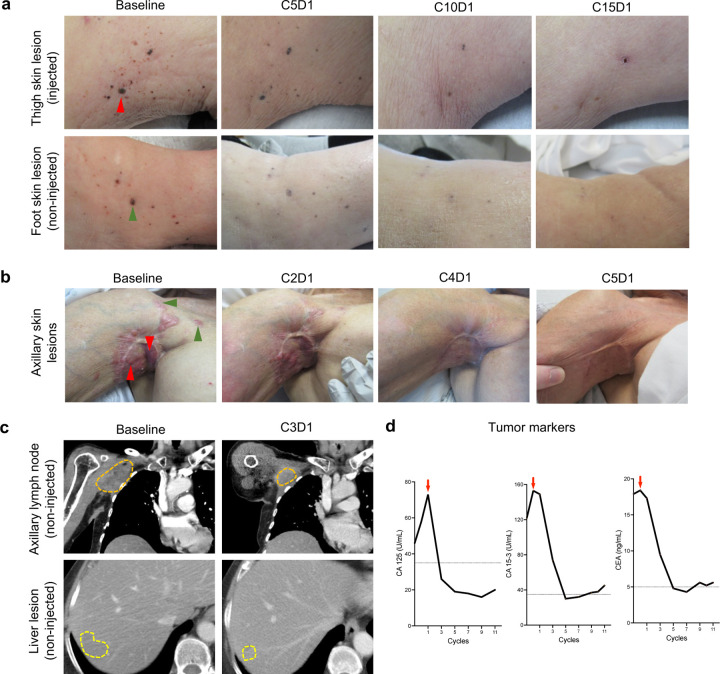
Clinical characterization of complete responders to 2141-V11. a, Representative photographs of the cutaneous lesions of patient 06 that achieve CR to 2141-V11. Images of injected (red arrows) and non-injected target lesions (green arrows) were obtained at baseline and at indicated cycle and day timepoints. b, Representative photographs of patient 14 that achieve complete response to 2141-V11. Images of injected (red arrows) and non-injected target lesions (green arrows) were obtained at baseline and at indicated post-treatment timepoints. c, Radiographic assessment of non-target axillary lymph nodes and liver metastases were obtained at baseline and after treatment with 2141-V11 in patient 14. d, Tumor markers of patient 14 before and after treatment with 2141-V11, red arrows indicate time of treatment initiation. Dashed lines indicate normal values of each tumor marker. C: Cycle. D: Day.
